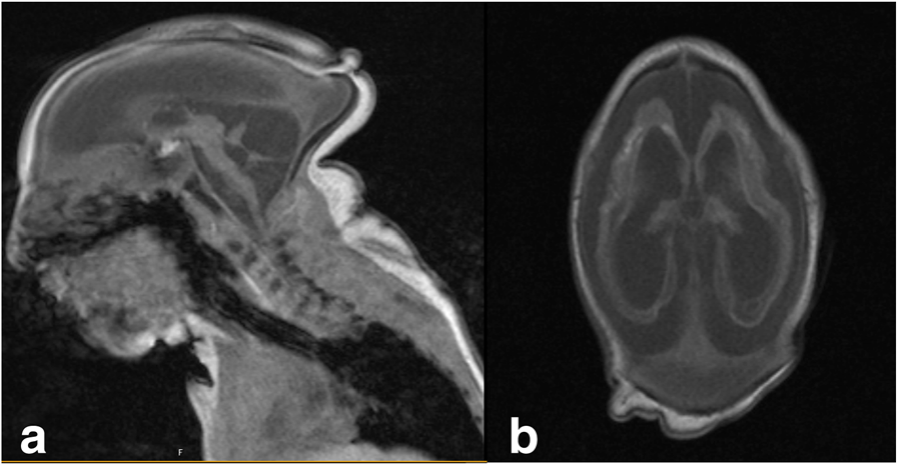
a Neonatal brain magnetic resonance imaging T1-weighted image demonstrating grossly abnormal brain formation with diminished brain volume and diffuse lissencephaly in the supratentorial brain parenchyma. There is marked atrophy of the corpus callosum, cerebellum, and brainstem. b Neonatal brain magnetic resonance imaging T1-weighted image highlighting ventriculomegaly, increased T1 signal in the cortical-subcortical white matter junction at areas of dystrophic calcification with diffuse ventriculomegaly
